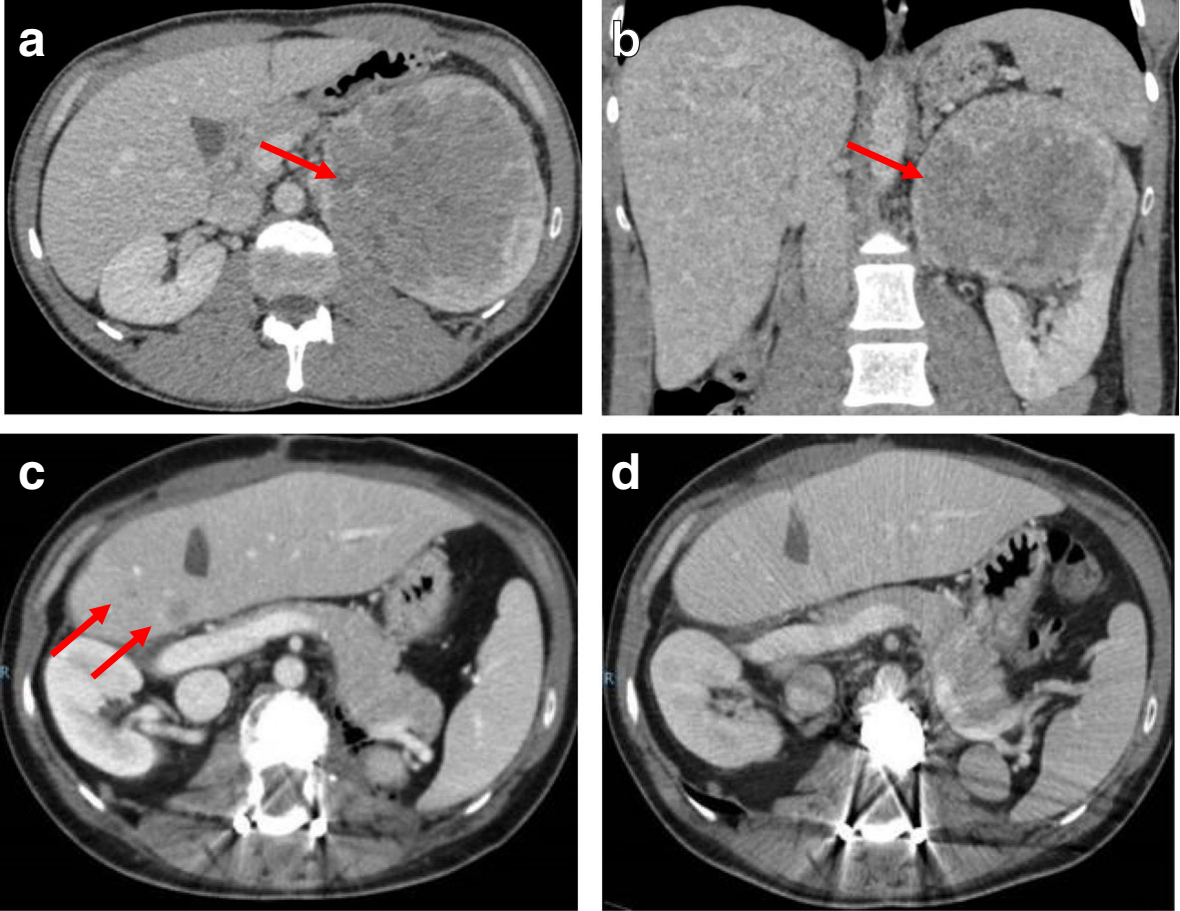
Computed tomographic (CT) scans. Left renal mass of 10 × 12 cm at diagnosis (a and b). Liver recurrence (c) and response after 5 months of sirolimus treatment (d)
